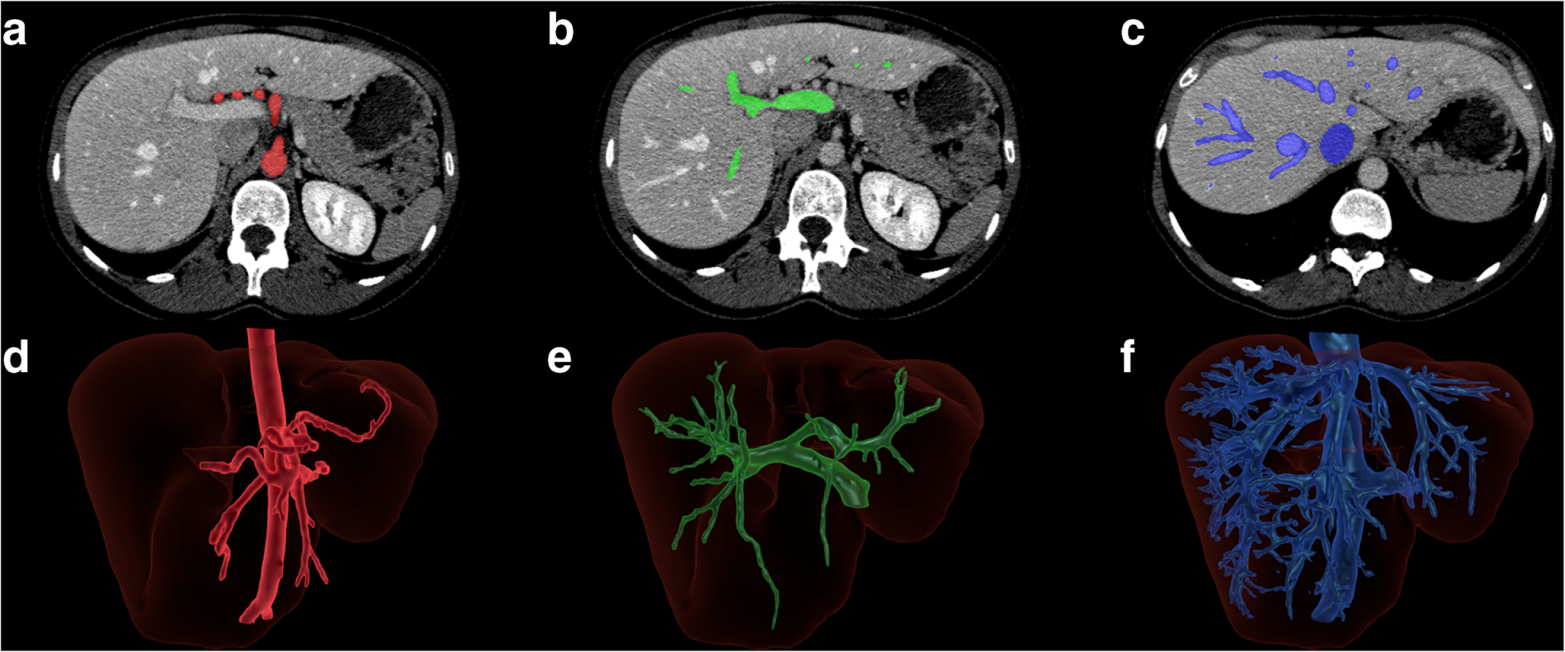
Liver subsegmentation according to vascular anatomy. Axial enhanced CT showing the segmented a hepatic arterial, b portal venous and c hepatic venous structures. Three-dimensional rendering of the same liver showing the corresponding segmented d arterial, e portal venous and f hepatic venous structures [13]
